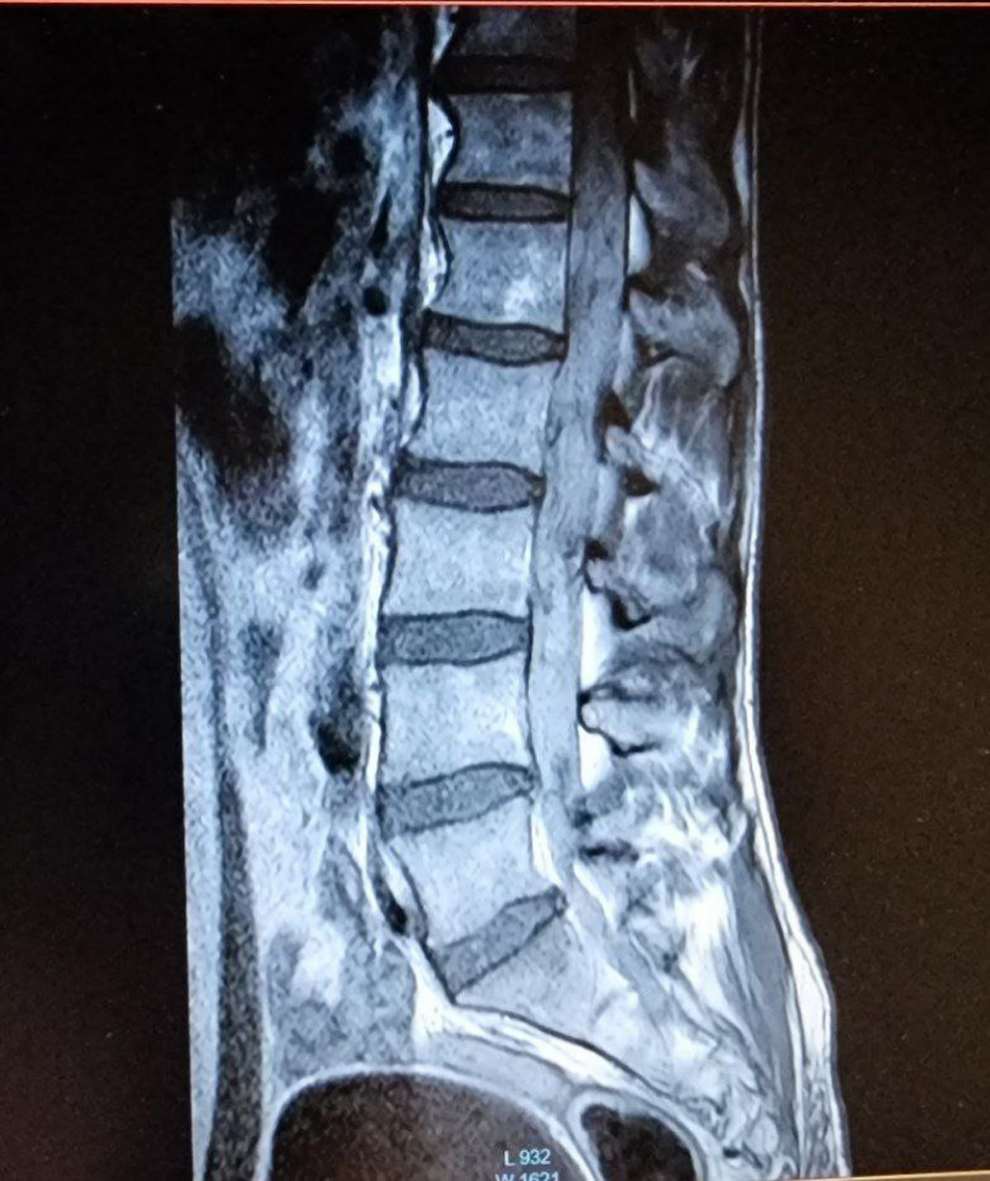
MRI of the lesion in the dorsal and lumbar columns of the spinal cord (T1).
